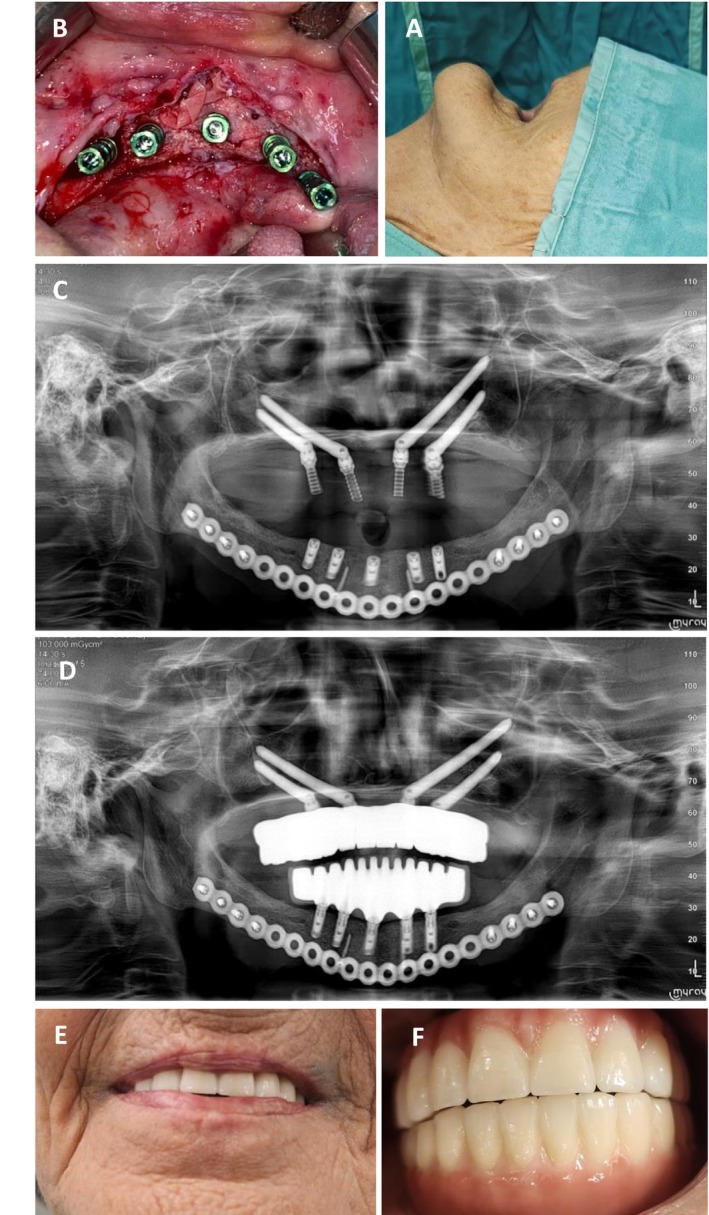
Complete implant‐supported reconstruction—Case 2. (A) Preoperative profile view showing the ill lip support by the atrophic jaws. (B) Intraoperative mandibular dental implantation procedure. (C) 1 week follow‐up panoramic radiograph demonstrating the positioning of the dental implants in the mandible and the zygomatic implants. (D) Panoramic radiograph demonstrating the final prosthodontic treatment. (E, F) Intraoral view of the final prosthodontic treatment demonstrating the functional and esthetic rehabilitation following complete implant‐supported reconstruction.
